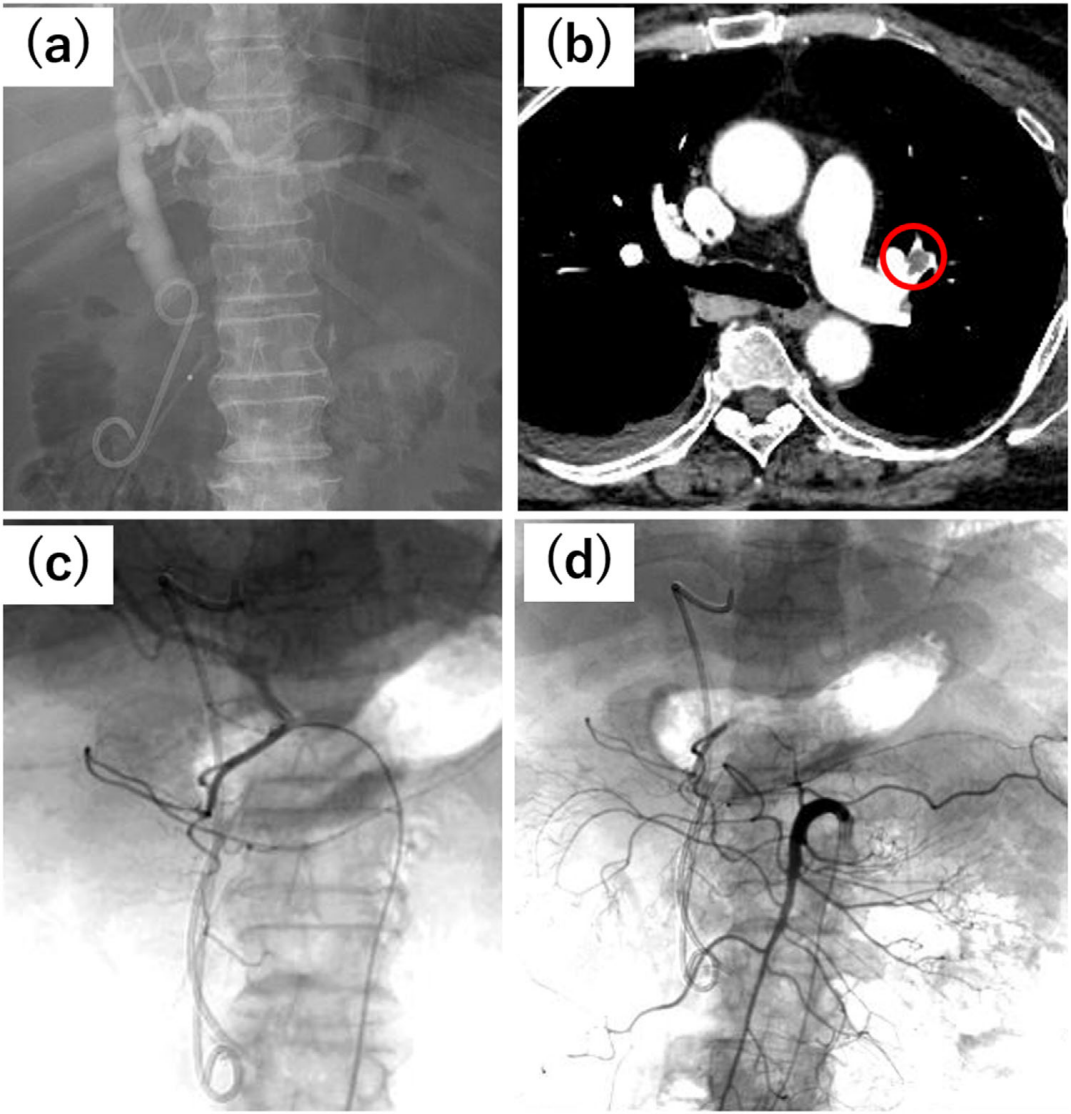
(a) We placed 7‐French (Fr) double pigtail and 5‐Fr straight stents for the biliary and pancreatic duct obstruction, respectively. (b) Contrast‐enhanced computed tomography revealed a thrombus in the left pulmonary artery (red circle). (c) Angiography of the gastroduodenal artery and (d) superior mesenteric artery, showing no pseudoaneurysm or extravasation
